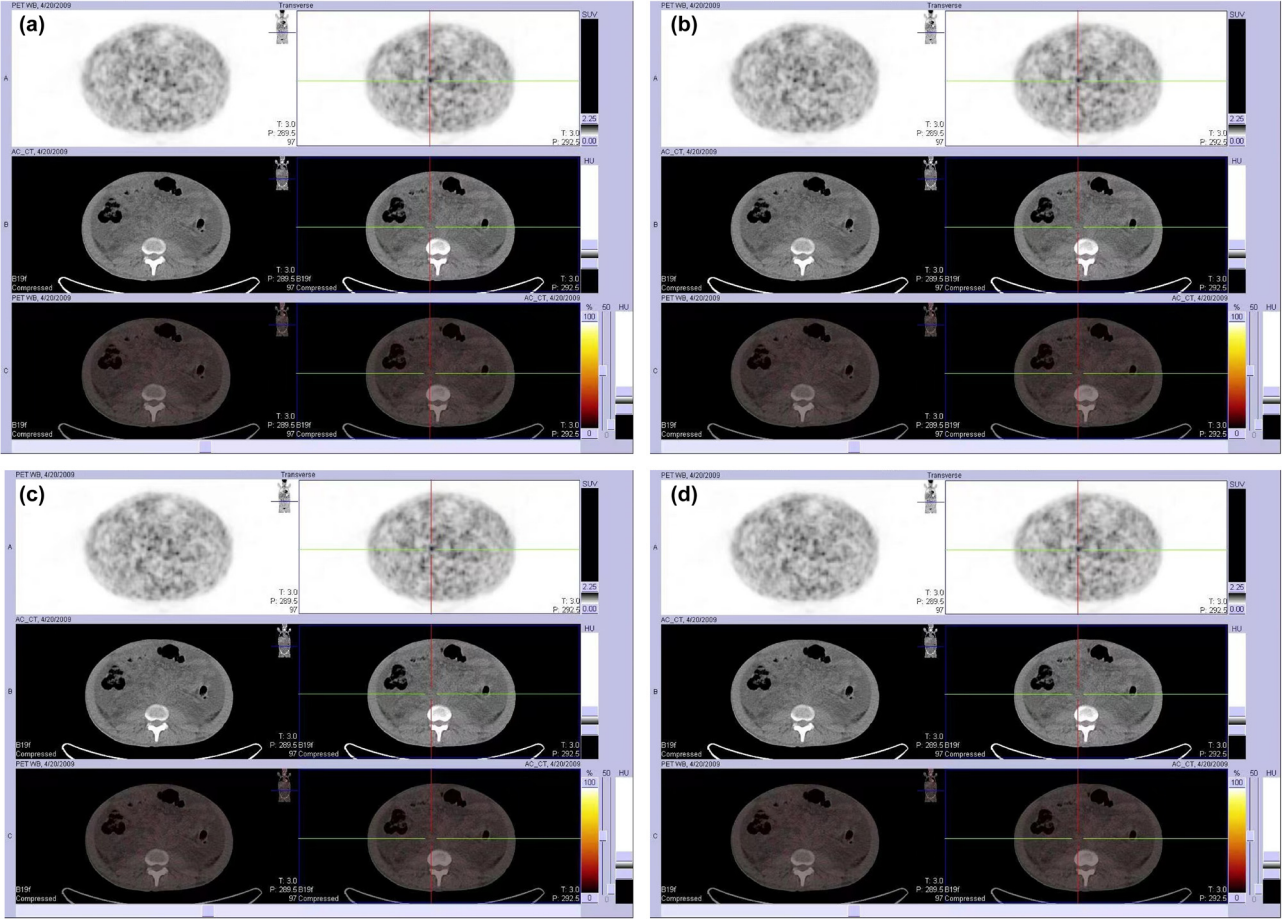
Fluorodeoxyglucose (FDG)-PET/CT scan at initial presentation revealed a mild abnormal accumulation of FDG in bilateral pleural effusion, as well as ascites, and multiple small lymph nodes (located behind the 12th thoracic and 4th lumbar vertebrae, with a diameter between 4 and 9 mm) (maximum standard uptake value: 1.8–2.2).
